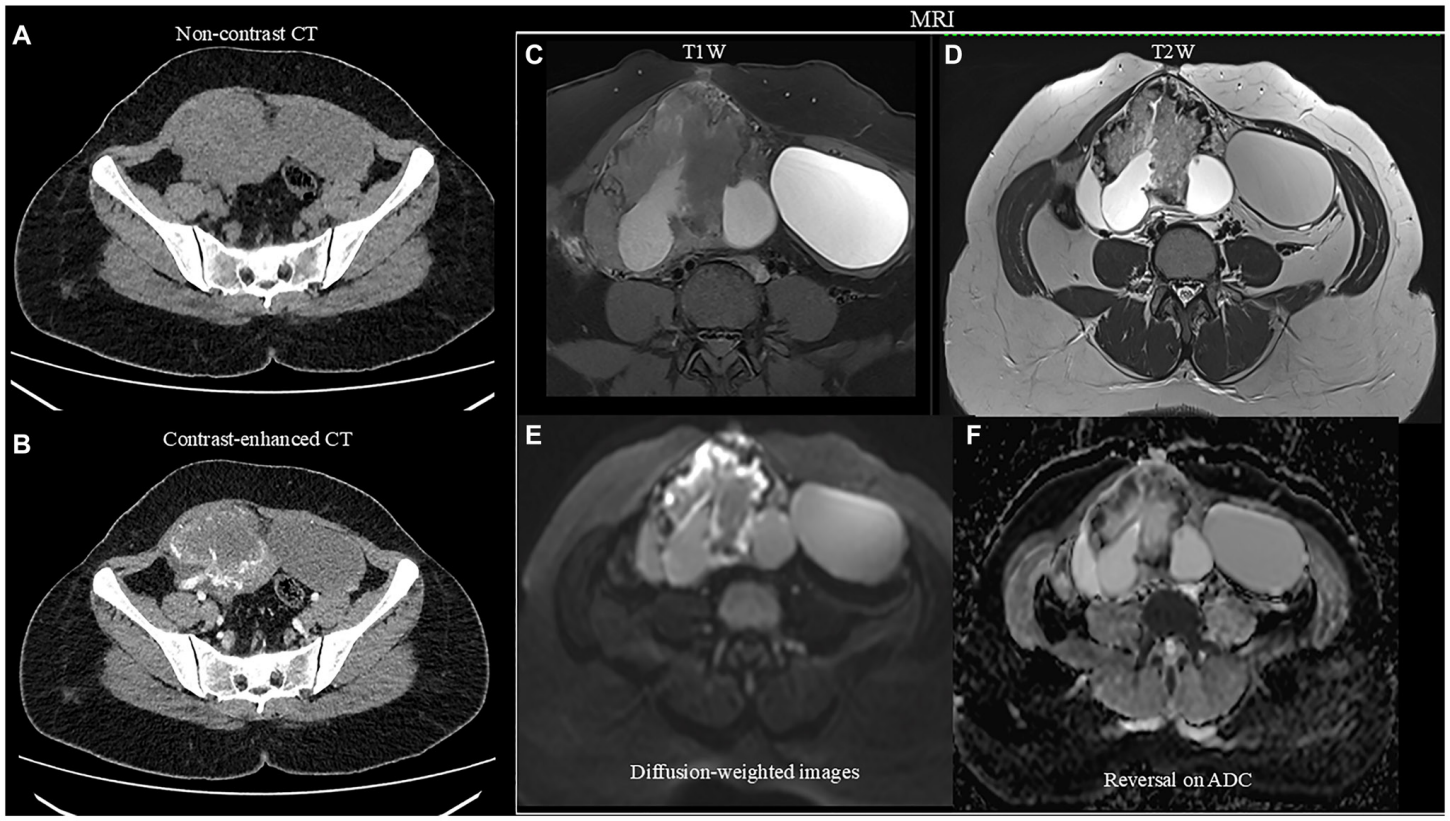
(A, B) Non-contrast and contrast-enhanced CT images demonstrating a large, solid-cystic lesion arising from the right adnexa. No calcifications were seen on the non-contrast images. Following contrast administration, an intense peripheral enhancement of the solid component was observed, predominantly supplied by a large vascular pedicle originating from the right uterine artery. The right ovary is not visualized separately from the lesion. Additionally, a large cystic structure is noted in the left adnexa; (C) Fat-suppressed T1-weighted images revealing a solid-cystic lesion in the right adnexa, with the solid component demonstrating intermediate signal intensity and the cystic areas appearing hyperintense, suggestive of hemorrhagic content. Additionally, a hyperintense cyst was noted arising from the left ovary; (D) On T2-weighted images, the solid component of the lesion demonstrates peripheral hypointensity and central intermediate signal intensity, while the cystic component appears hyperintense. The left ovarian cyst shows intermediate signal intensity, suggestive of internal hemorrhage; (E) Diffusion-weighted image revealing an undulating peripheral area of diffusion restriction within the solid lesion; (F) Showing reversal on Apparent Diffusion Coefficient (ADC), favoring neoplastic etiology.
